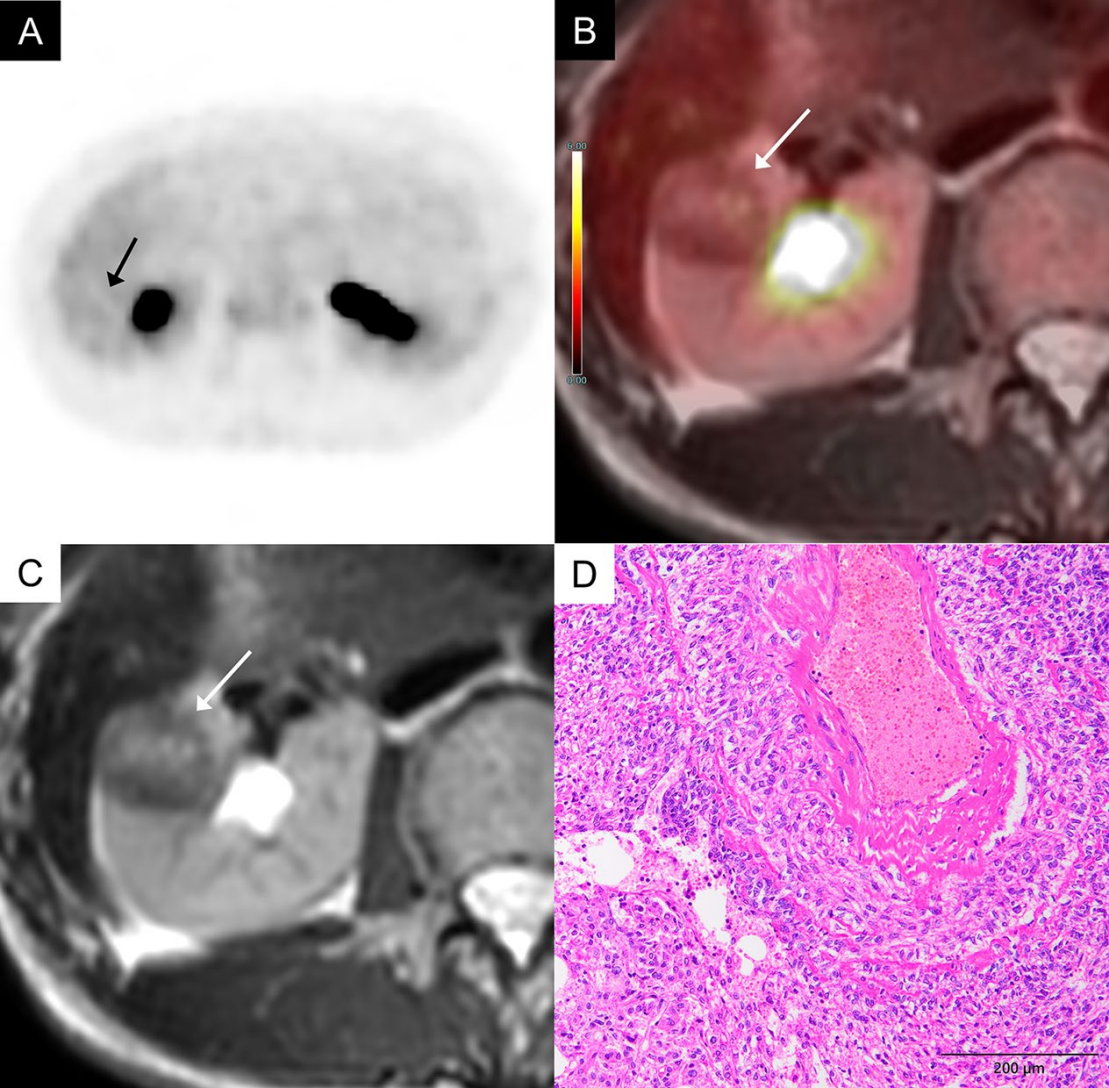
A 44-year-old woman with an angiomyolipoma of the right kidney. A In the FDG-PET image (grayscale), the uptake of the right renal tumor (arrow) is almost the same as that of the renal cortex, making tumor identification difficult. B Axial FDG-PET/MRI image shows FDG uptake in the right renal mass (arrow, SUV max: 2.49) comparable to that of normal renal cortex (SUV max: 2.08). C Axial T2-weighted image shows a low signal intensity in the mass (arrow). D The tumor consists of three components: smooth muscle cells, adipose tissue and vascular tissue. The smooth muscle cells have short spindle-shaped nuclei without high-grade morphology
